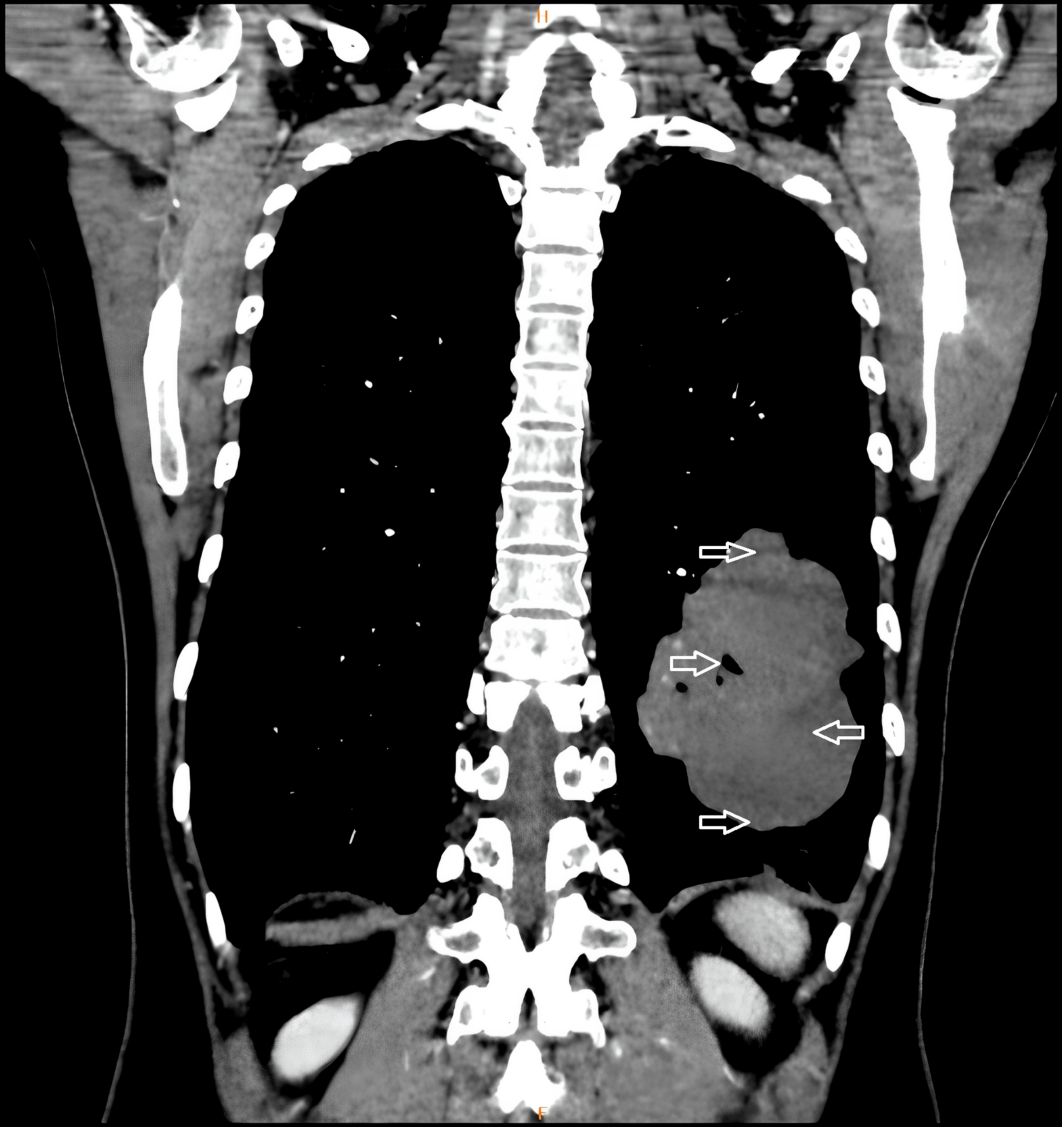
MRI of the lung showing a mass of 140 mm x 90 mm in the lower left lung.
